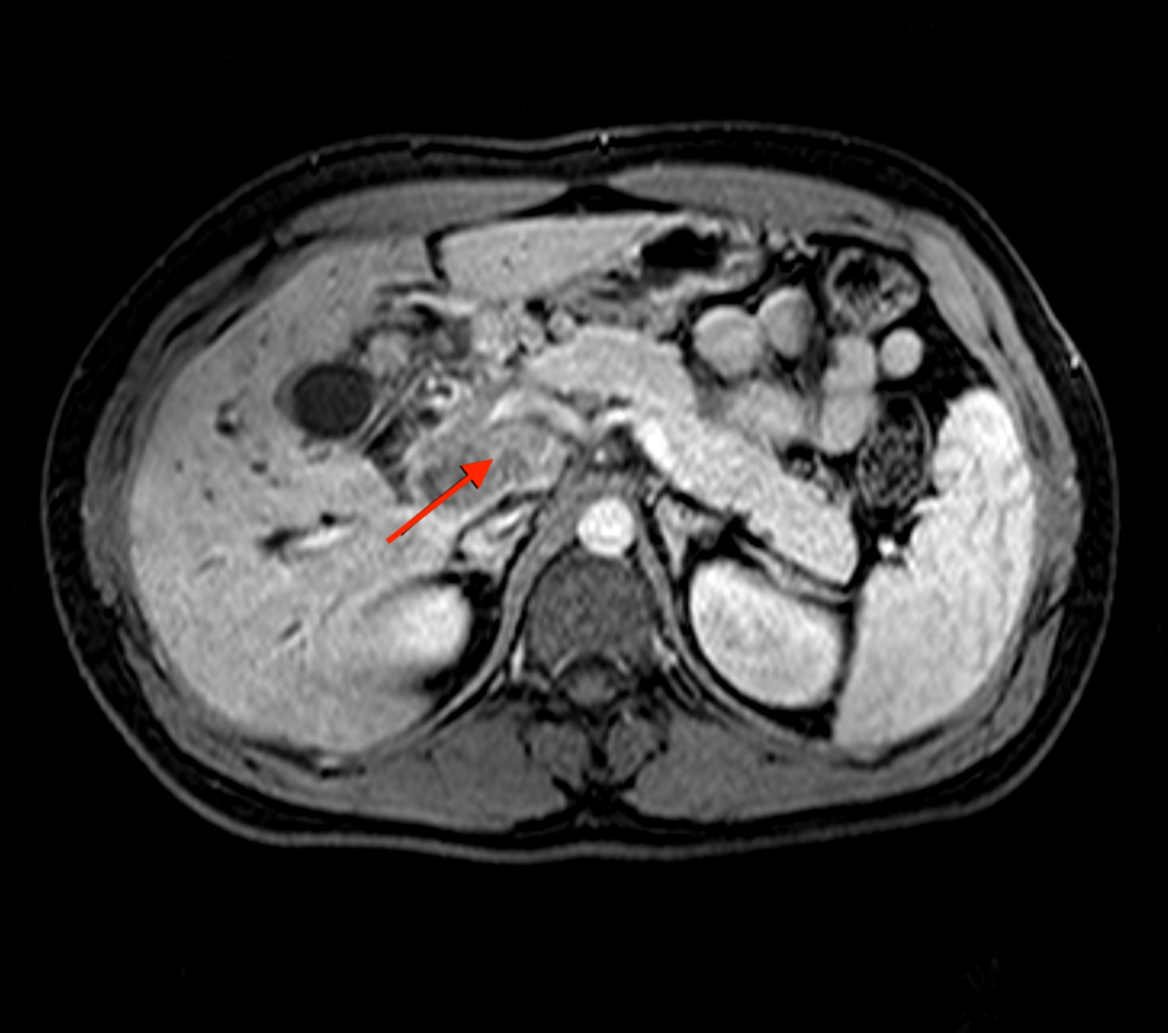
Abdominal MRI with gadolinium injection depicting an irregularly enhancing lobulated mass at the uncinate process of the pancreas measuring 2 cm x 1.9 cm.
